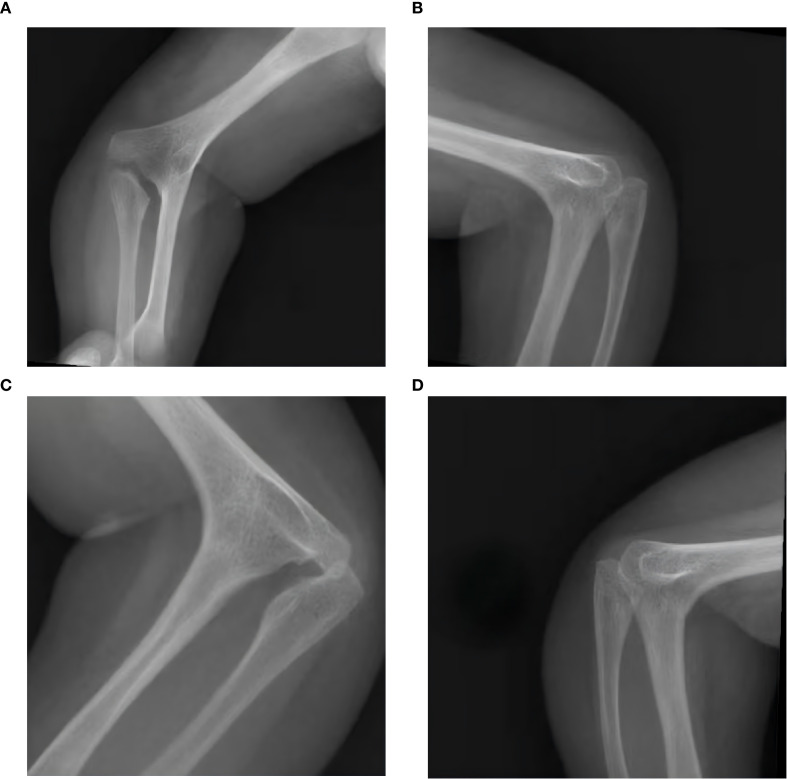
Bilateral elbow joint X-ray of the patient [(A) Anteroposterior view of the left elbow; (B) lateral view of the left elbow; (C) anteroposterior view of the right elbow; (D) lateral view of the right elbow].
